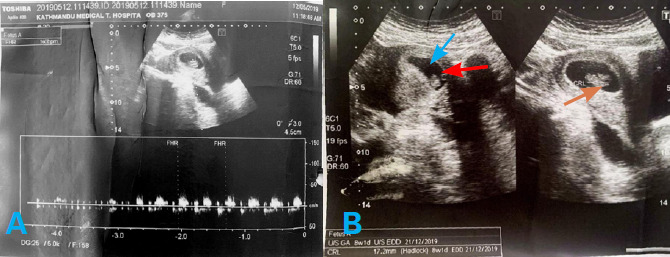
Transabdominal ultrasonography with pulsed wave Doppler interrogation. A) intrauterine gestation sac and embryonic pole with a fetal heart rate of 160 bpm, B) intrauterine gestation sac (blue arrow) with single yolk sac (red arrow) and single embryonic pole of 17.2mm (orange arrow).
